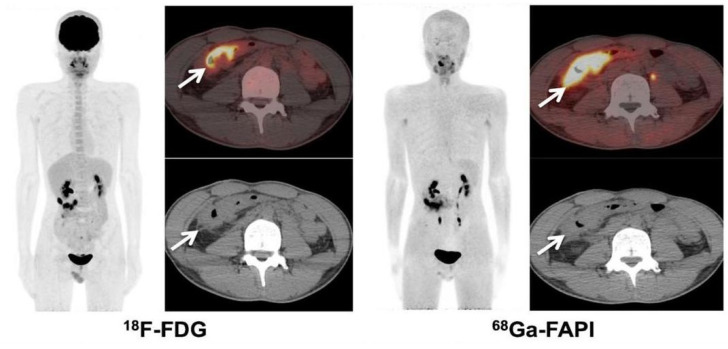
^18^F-FDG PET/CT showed hypermetabolism in the intestines around the anastomosis in the right upper quadrant of the abdomen (SUVmax 11.4). The involved bowels also had intense uptake of ^68^Ga-FAPI (SUVmax 14.1). The arrow shows high metabolic uptake of the intestinal tract around the anastomotic site after Crohn’s disease. Adapted from Ref. [71].
